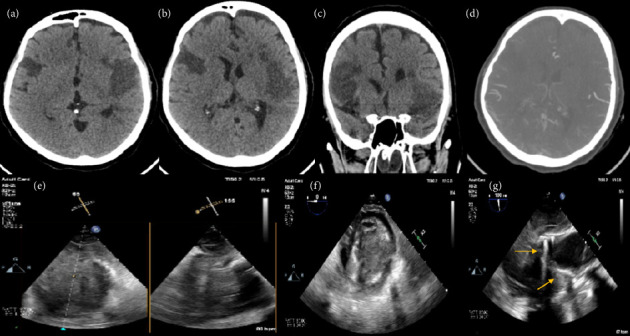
CT brain (a–d) and TEE (e–g) images of a 55-year-old woman developed postcardiotomy cardiogenic shock and required central VA-ECMO implantation. Despite left ventricular venting and anticoagulation, she developed thrombosis of the left ventricle and aortic root (e and f) with a stuck aortic valve (g, yellow arrows). NIRS continuous monitoring showed an abrupt decrease > 30%, emergency CT brain revealed bilateral ischemic infarcts (a–c), and angiography revealed patent middle cerebral arteries with paucity of the distal branches at areas of strokes (d).
